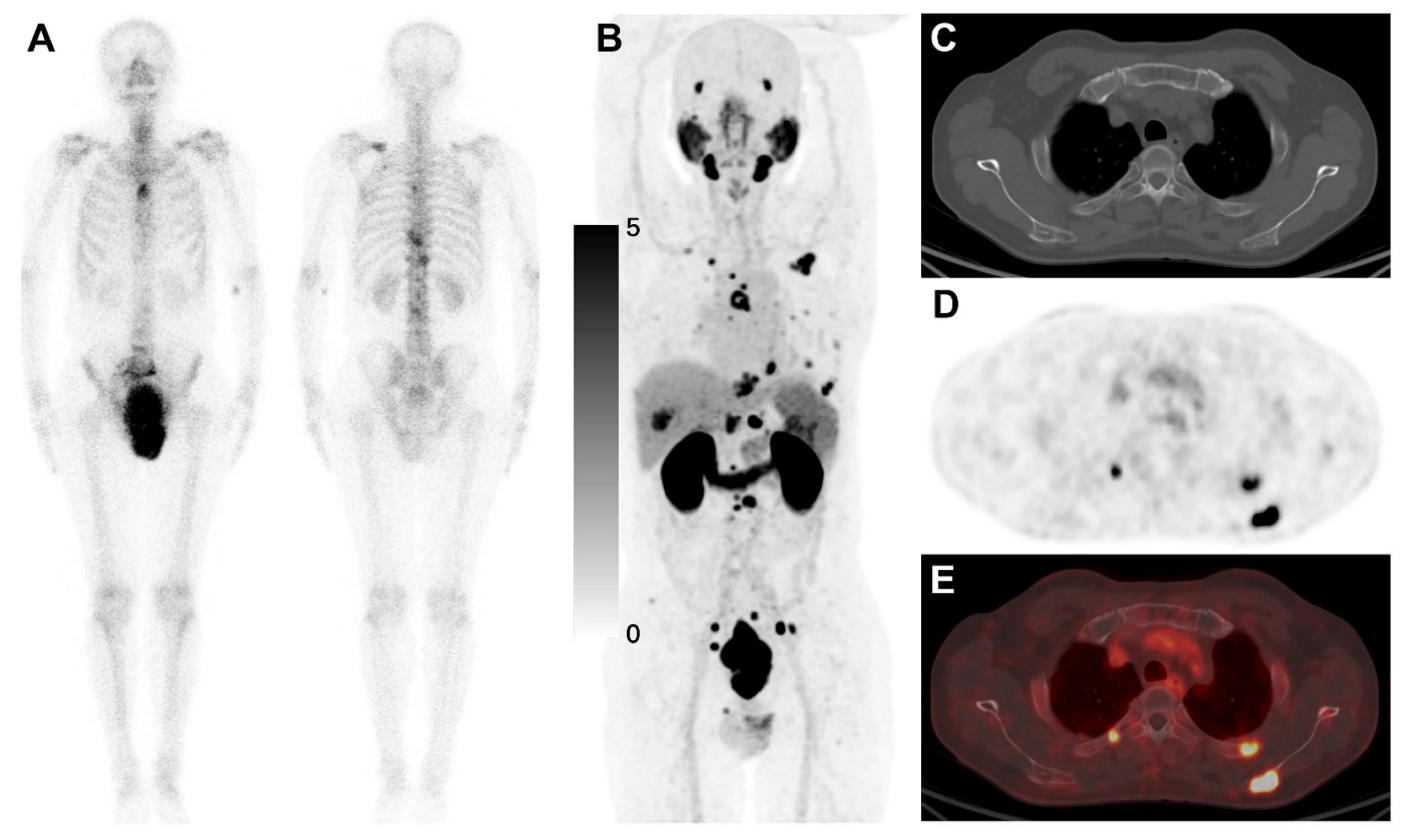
A 64-year-old man with PCa underwent a bone scan and [^18^F]AlF-Thretide PET/CT within three days. Prior to the [^18^F]AlF-Thretide PET/CT scan, his total PSA level was 540 ng/mL. (A) The bone scan identified 9 bone metastases. (B-E) However, the [^18^F]AlF-Thretide PET/CT revealed 26 bone metastases with its superior imaging capabilities. These additional lesions, not detected on the bone scan, highlight the increased sensitivity of [^18^F]AlF-Thretide PET/CT. The enhanced resolution and higher affinity of [^18^F]AlF-Thretide for PSMA-expressing tissues allowed for the identification of smaller and more subtle metastatic foci, underscoring the potential of this imaging modality to provide a more comprehensive staging in PCa.
